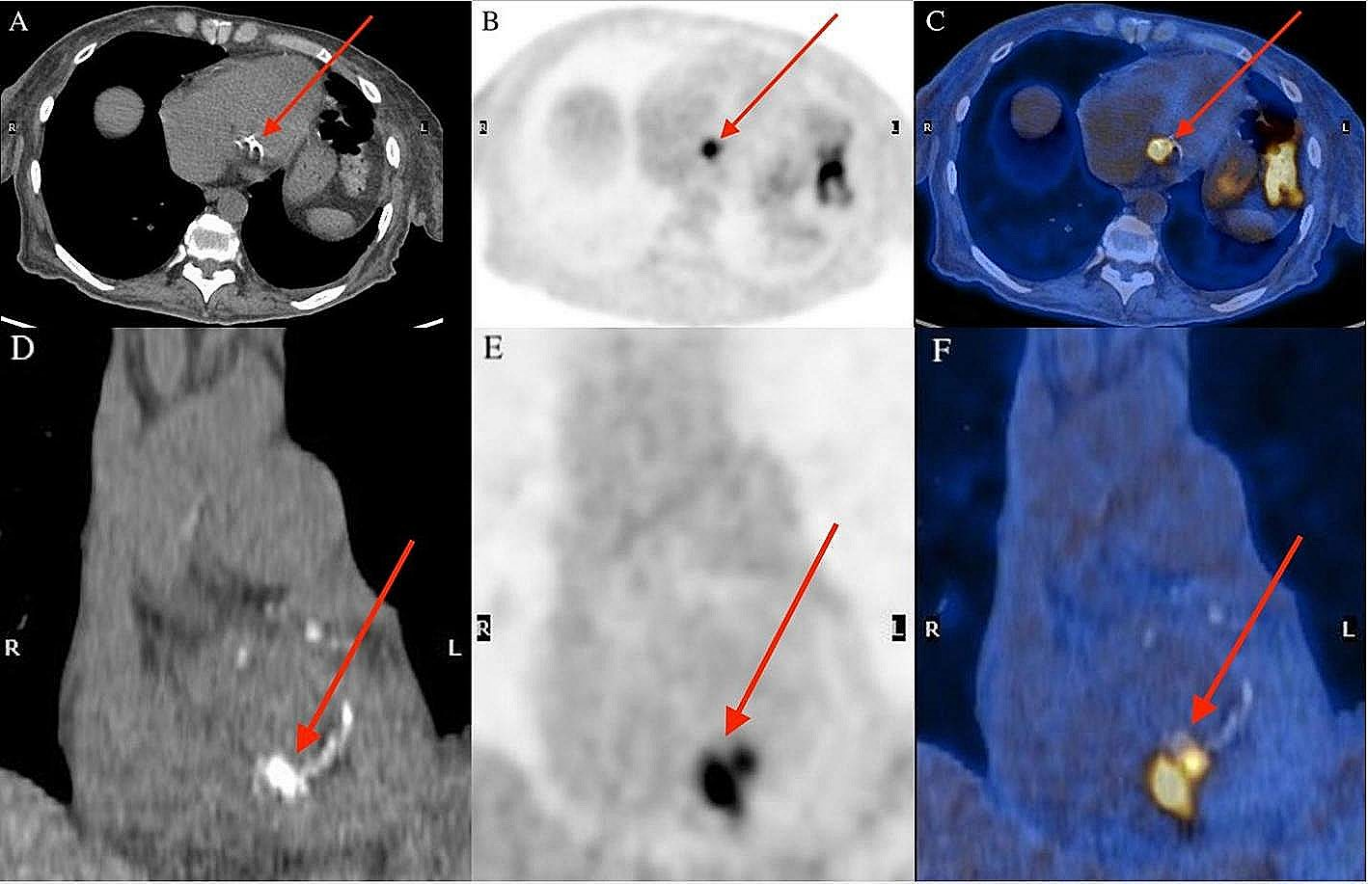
A-F, ^18^F-FDG whole-body PET/CT was performed to evaluate for a possible infectious etiology of the mobile right atrium mass visualized on TTE. The study revealed intense, focal FDG uptake (SUVmax 6.1) at the calcified mitral valve, consistent with mitral valve infective endocarditis (A-F, arrow). No FDG-avid lesion was observed in the right atrium corresponding to the mobile mass described on TTE.
